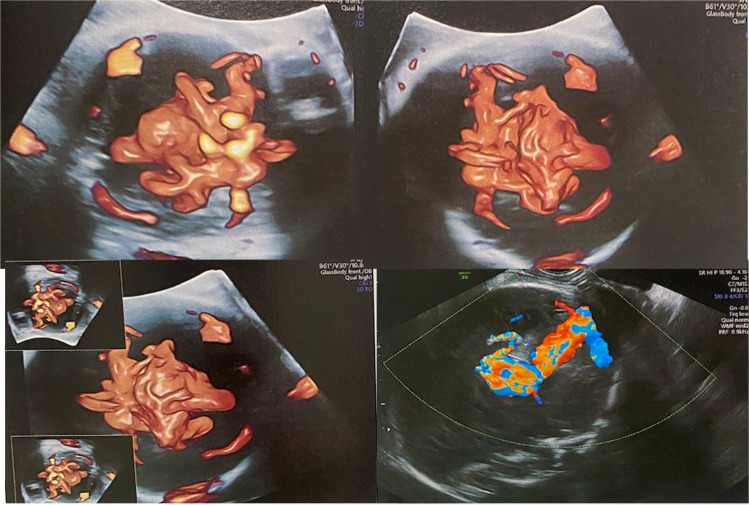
Obstetric Doppler ultrasonography demonstrating an aneurysmal dilation of the vein of Galen (present-case images)
